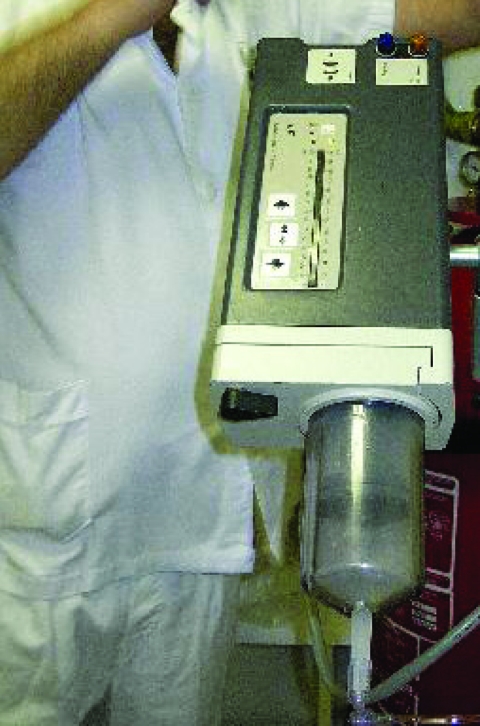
Equipment for contrast administration in computed tomography scan.
